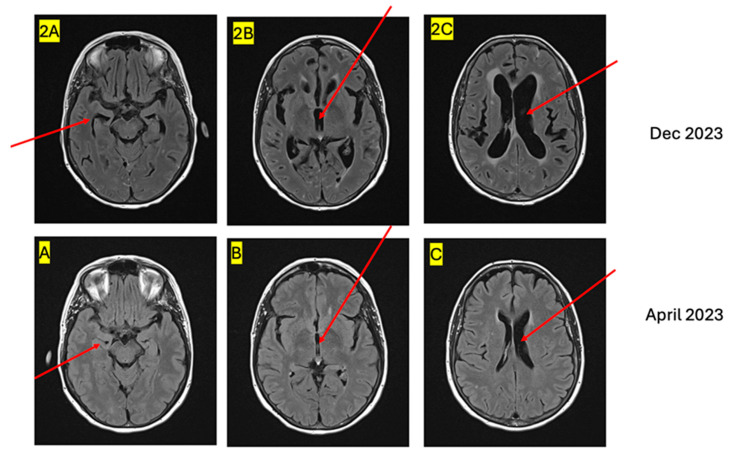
MRI FLAIR image demonstrating severity of hydrocephalus. (A,2A) Comparing the caliber of temporal horns; (B,2B) comparing caliber of the third ventricle; (C,2C) comparing caliber of the lateral ventricles).
